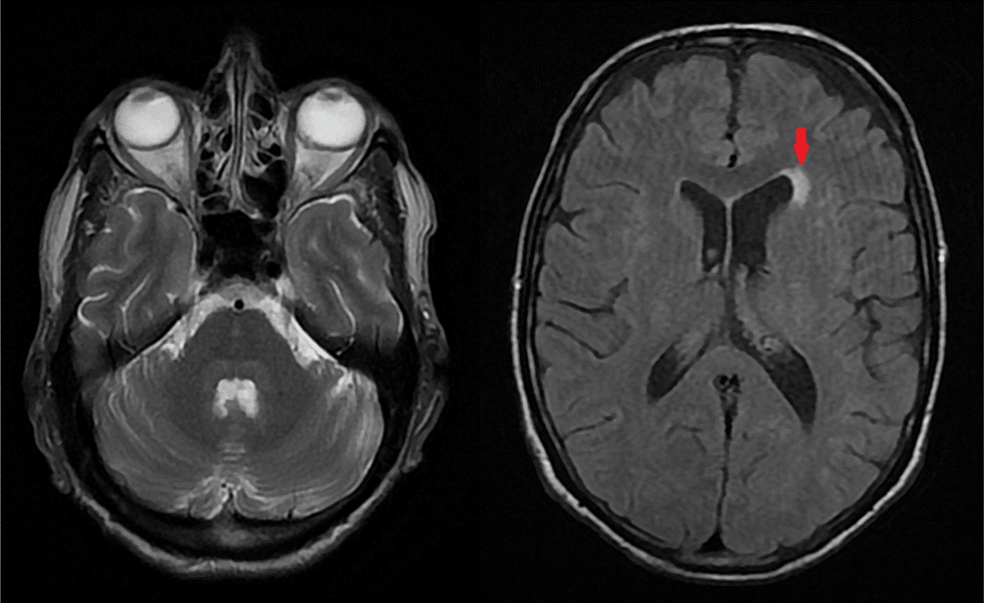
Cerebral MRI showing some vascular hyperintensities with no lesion in the pons
